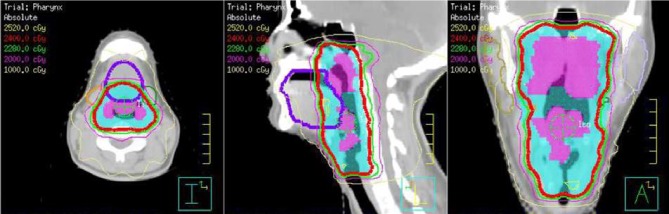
CT scan of the neck with contrast. Clinically involved tissues are shown in pink and the radiation planning target volume is shown in blue. The isodose lines show coverage of the target with 24 Gy and sparing of the salivary glands.
